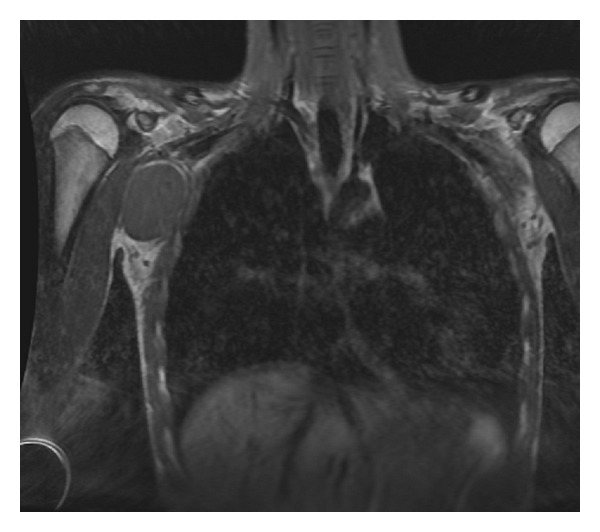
Coronal T1-weighted (500/22 [TR/TE msec]) image of the chest shows a well-defined oval mass of homogeneous signal intensity within the subcutaneous fat of the left axilla.
